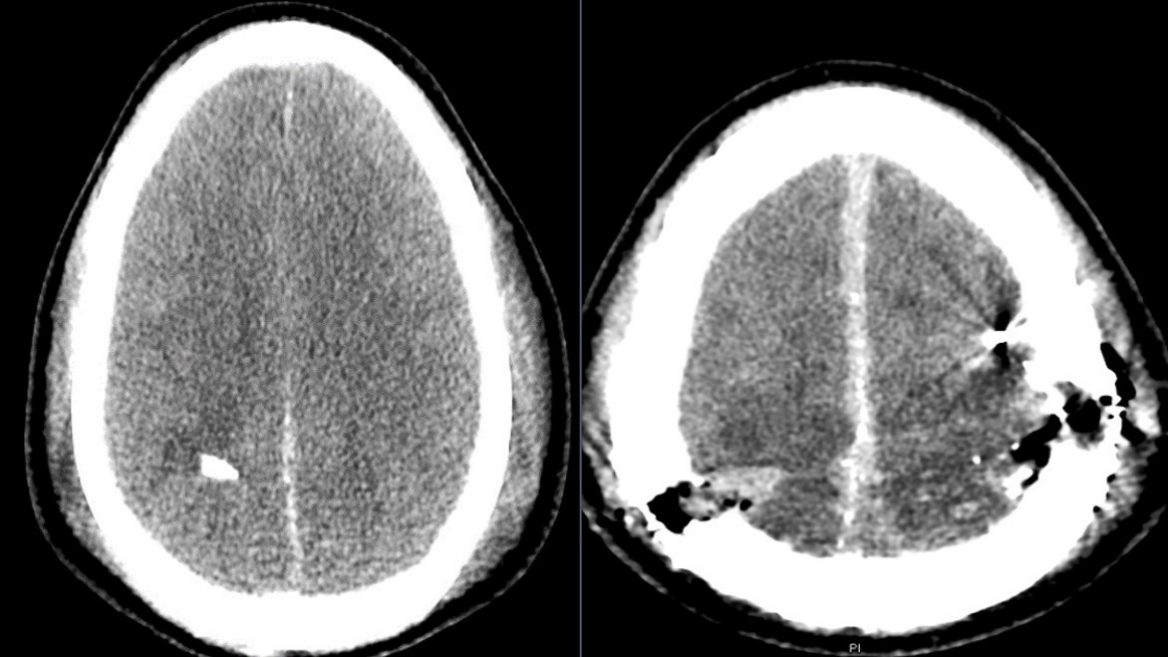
Computed tomography (CT) head revealed multifocal hemorrhagic contusions and edema along the cerebral convexities, complex and nondisplaced skull fractures along the projectile trajectory from the right to left parietal skull. The retained high-velocity projectile was in the left parietal bone.
